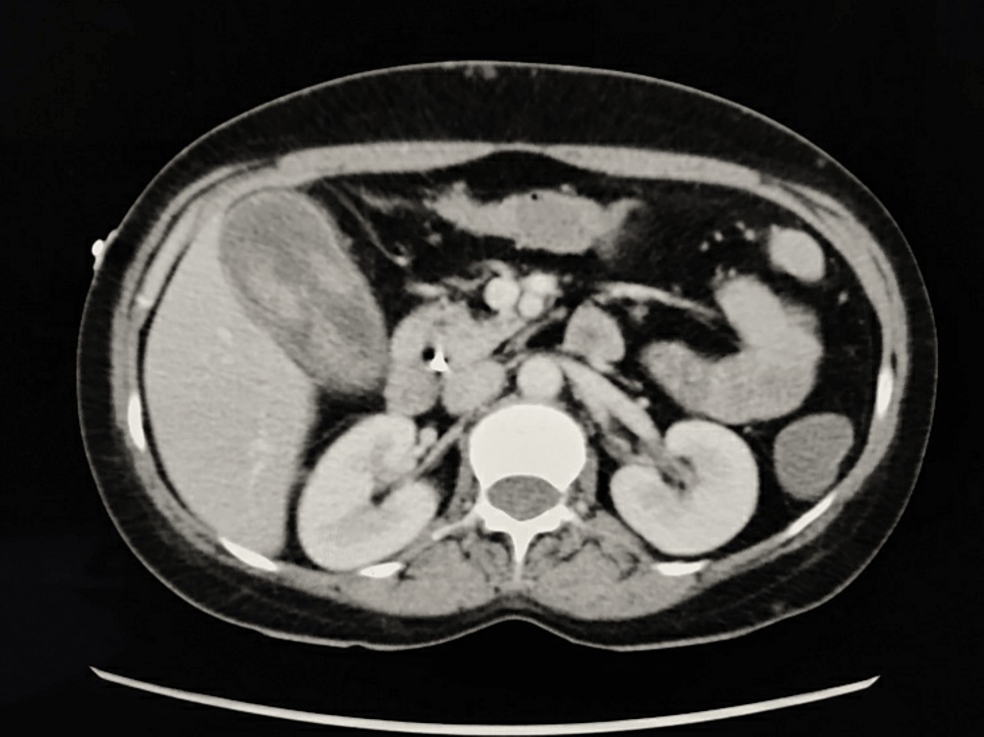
CECT abdomen image showing the gall bladder with multiple iso- to mildly hyperdense non-enhancing lesions – likely gall bladder sludge/calculi CECT: contrast-enhanced computed tomography
